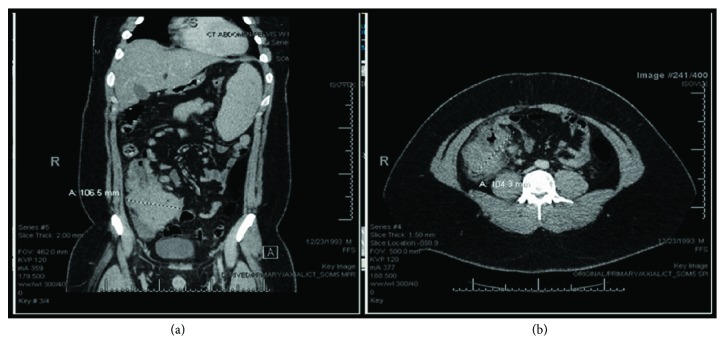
CT scan of the abdomen ((a) axial section, showed large 10 × 10 cm ileocolic mass, lymphadenopathy at the right colic area, and splenomegaly; (b) horizontal section).
